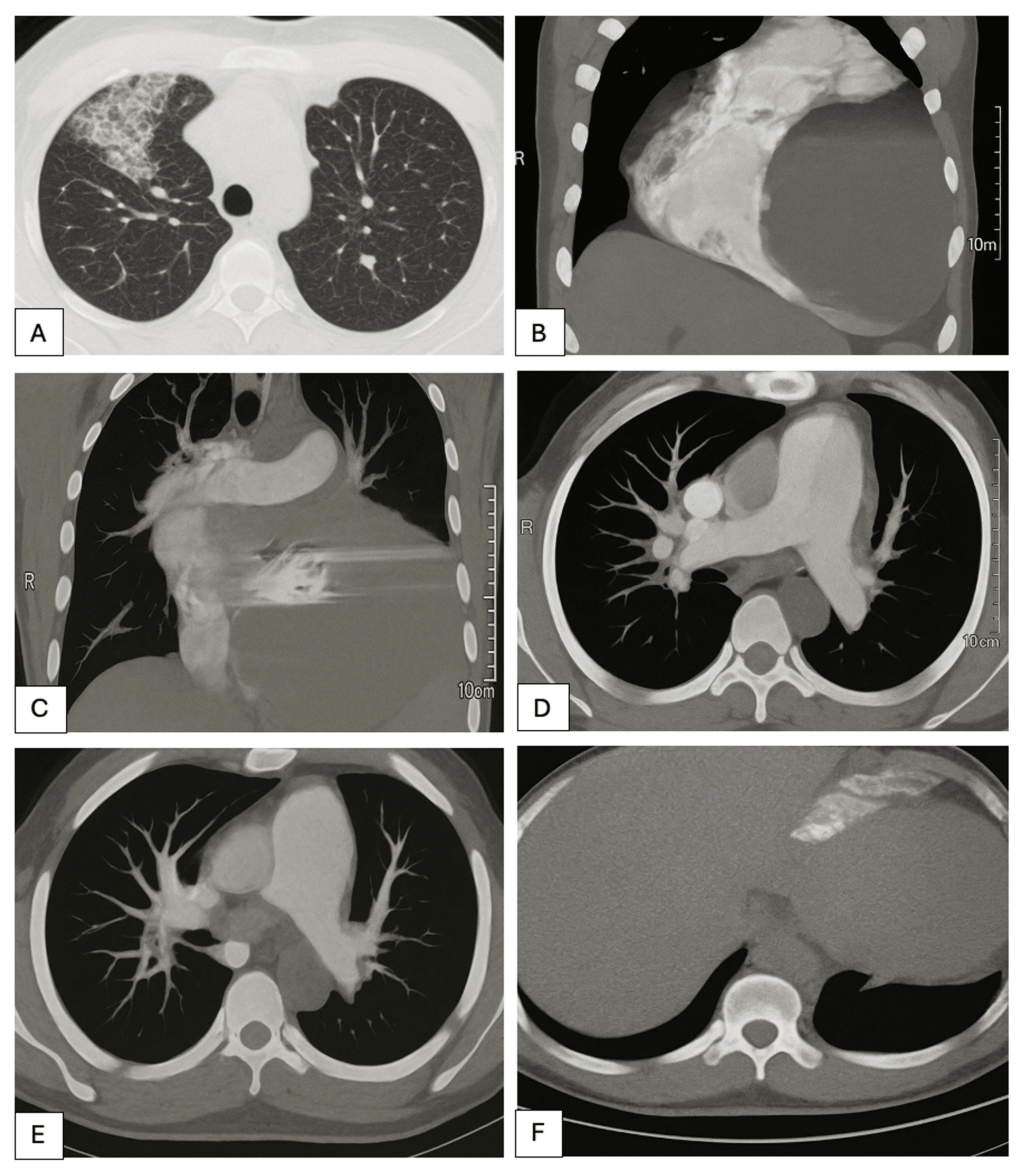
Computed tomography pulmonary angiogram performed two months prior to the death of the deceased patient. A. There was evidence of ground-glass opacity in apico-anterior segment of upper lobe of right lung, likely alveolar haemorrhages. B. Gross cardiomegaly was noted with maximally dilated left ventricle. Left atrium was also dilated. C. Prosthetic cardiac valves were seen in situ. D. There was dilatation of main pulmonary artery, as well as left and right pulmonary arteries suggesting pulmonary hypertension. No filling defect was seen. E. Segmental and sub-segmental pulmonary arteries also did not show any filling defect. There was no evidence of thromboembolism. F. Inferior vena cava was prominent.
